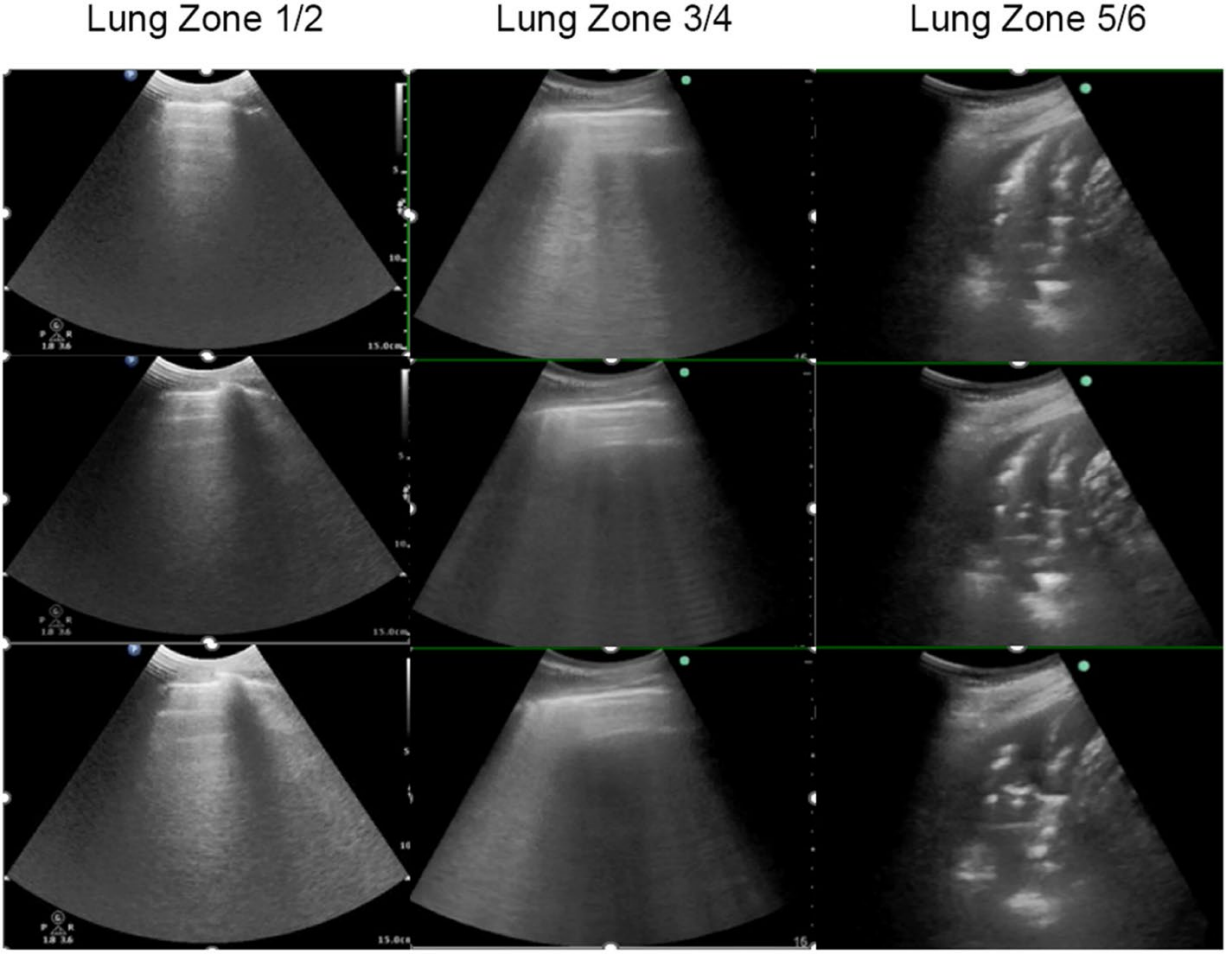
Lung ultrasound on admission
